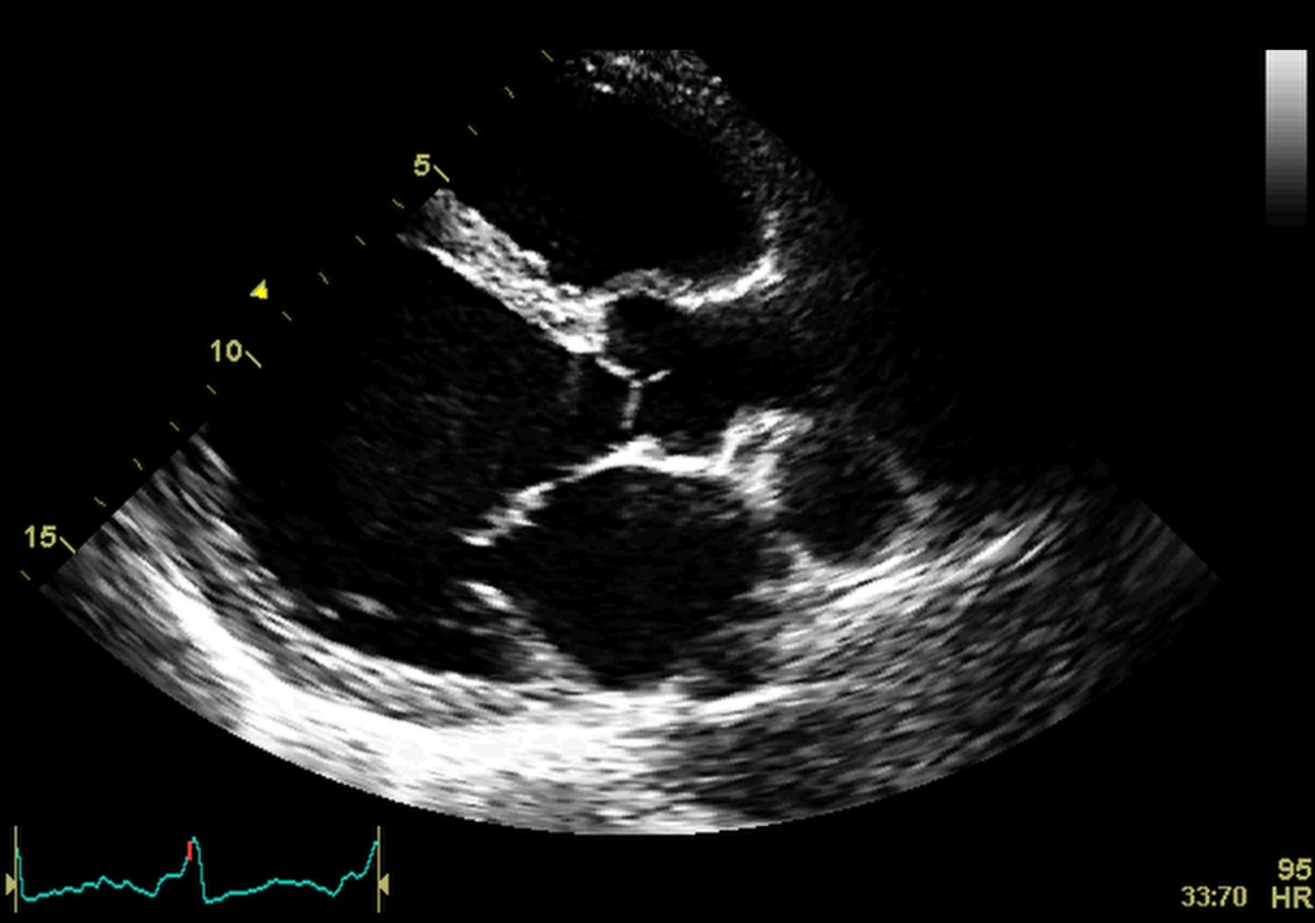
Echocardiographic aspect of DCM. Parasternal long-axis view
